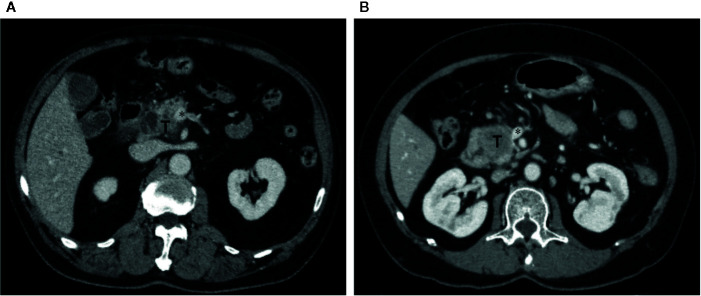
Representative cases which were reclassified after Radscore assistance. (A) Axial portal venous phase CT image in a 73-year-old man with PDAC in the pancreatic head. The vein was suspiciously deformed (*). The Radscore prediction gave a high probability of PV-SMV invasion. The interpretation of deformity of the vein between unassisted and Radscore-assisted was discrepant for both radiologists, they assigned PV-SMV invasion category with Radscore assistance. Intraoperative exploration confirmed the diagnosis of PV-SMV invasion. (B) Axial portal venous phase CT image in a 66-year-old female with PDAC in the pancreatic head. The vein was interpreted as teardrop shape by the junior radiologist (*) in first the reading. The Radscore prediction gave a low probability of PV-SMV invasion. The vein was interpreted as absence of teardrop shape by the junior radiologist (*) in the second reading with Radscore assistance, and PV-SMV non-invasion category was assigned. Intraoperative exploration confirmed the diagnosis of PV-SMV non-invasion.
